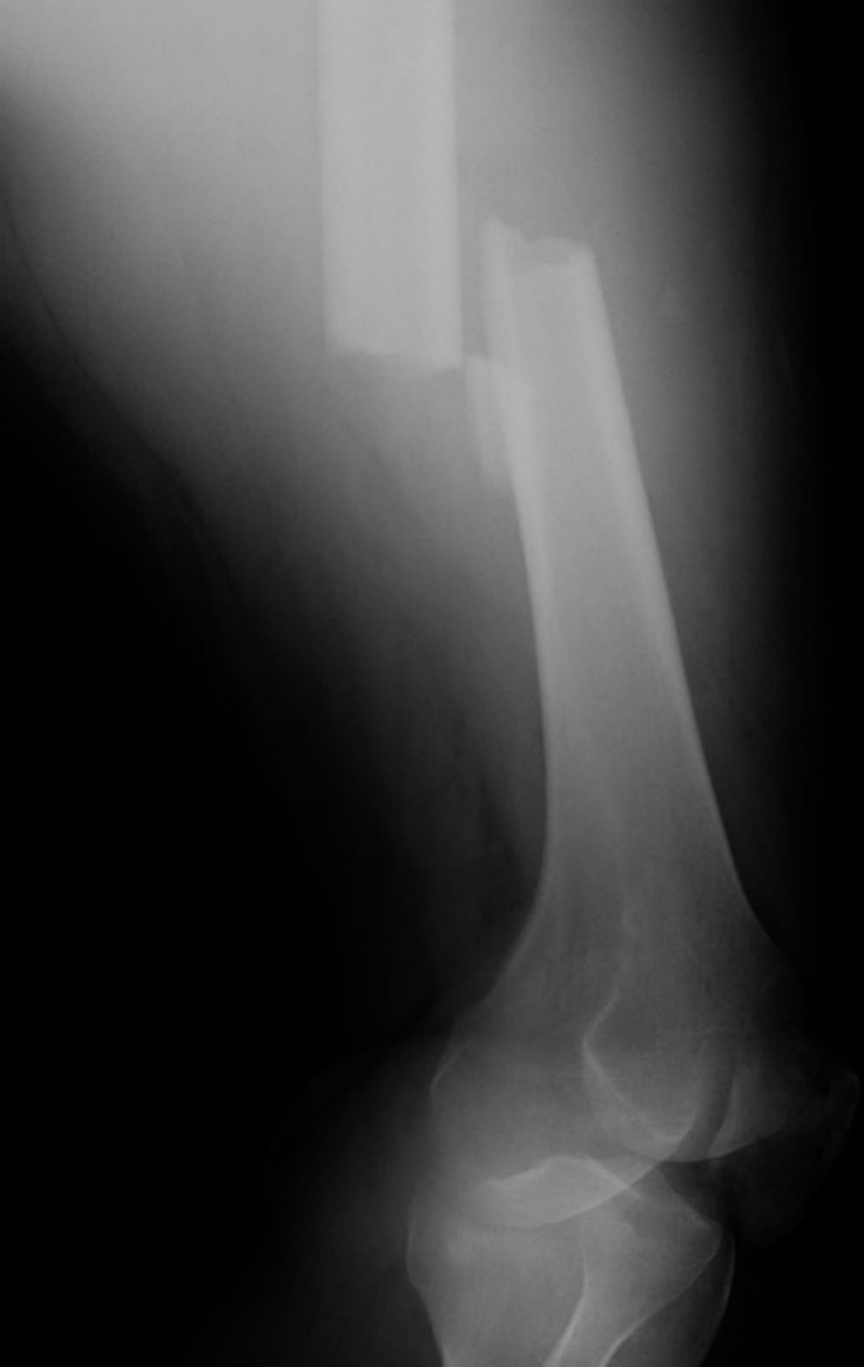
Plain radiograph of the left femur upon admission.
